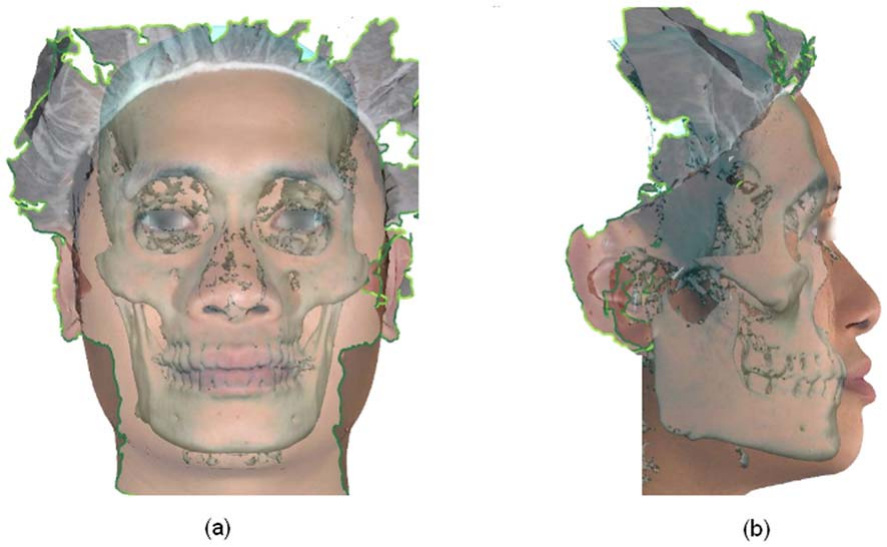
The frontal (a) and lateral (b) views of the virtual craniofacial model generated by fusing the Cone-beam CT and stereophotographic images.
